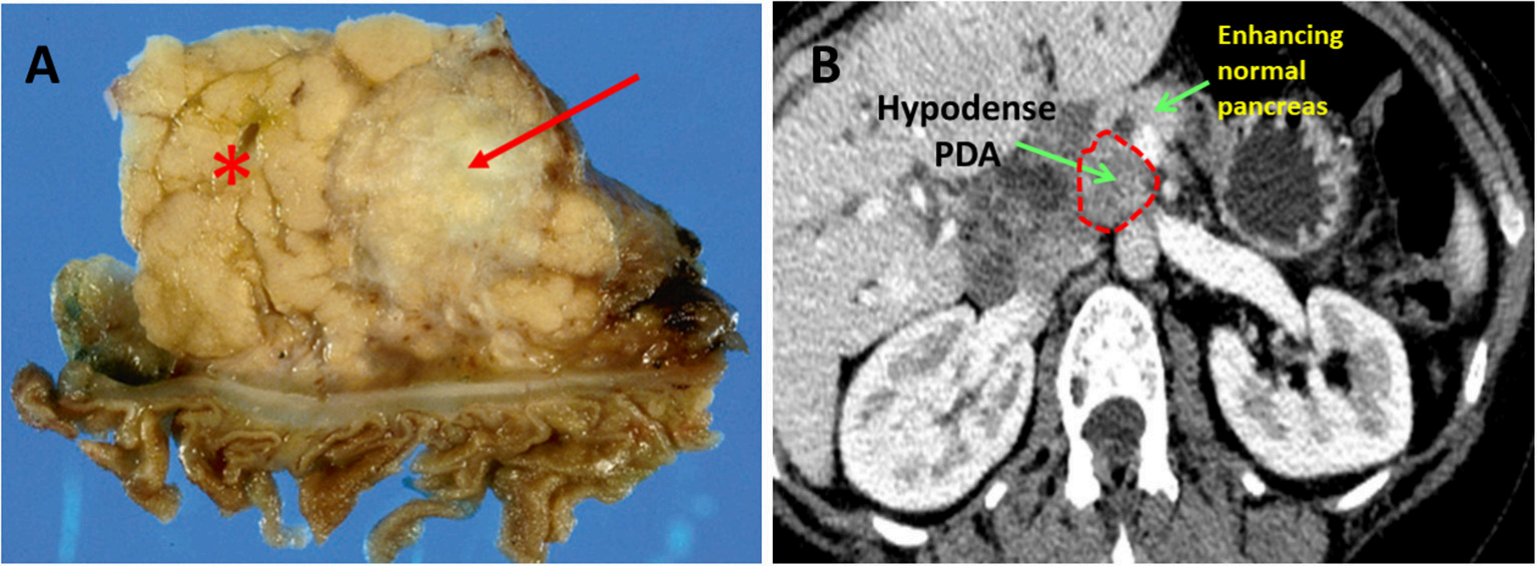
Pancreatic ductal adenocarcinoma. (A) Resected human PDA. The arrow identifies the characteristically pale, gray, and hypovascular pancreatic ductal adenocarcinoma. The asterisk marks pancreatic parenchyma. (B) On CT scan imaging with intravenous contrast, PDA appears hypodense (dark gray), while well-perfused normal pancreatic parenchyma shows bright enhancement due to penetration by the contrast.
